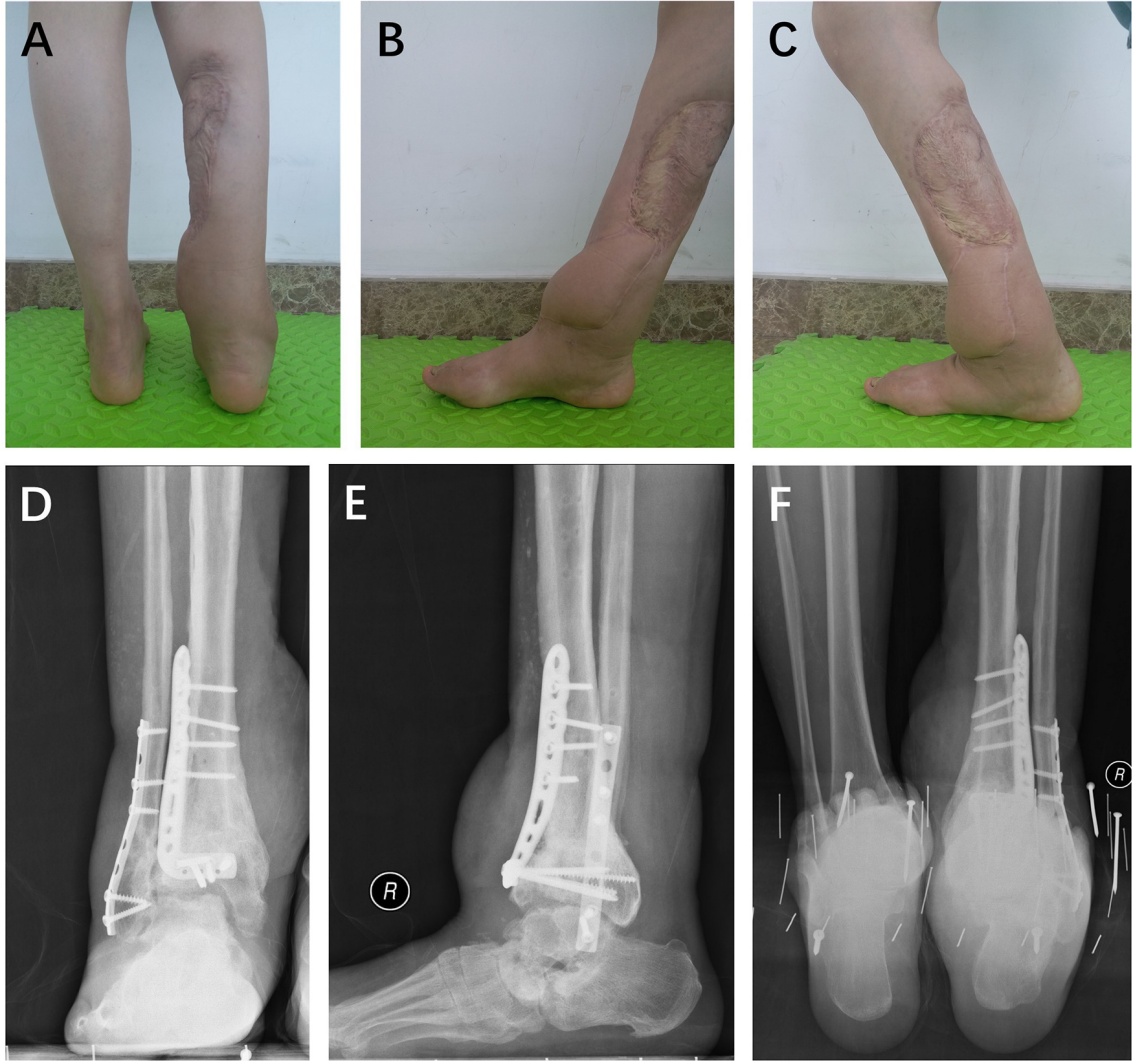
At the 18-month follow-up after the supramalleolar osteotomy, the patient displayed satisfactory hindfoot alignment (A) and range of motion (B,C). Charcot arthritis was detected at the Chopart joint (D,E), although no apparent symptoms were present. Radiographic images demonstrated the satisfactory hindfoot alignment (F).
